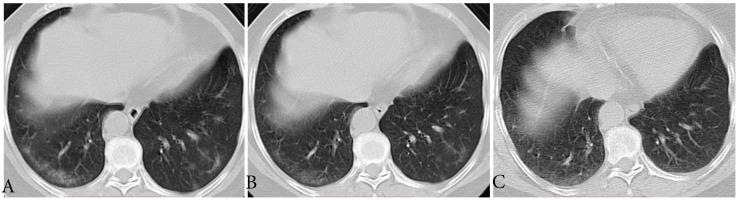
Typical evolution of type-GI in a 61-year-old female with asymptomatic SARS-CoV-2 infection. A, Day 0, the first chest CT showed multifocal lesions of subpleural GGO in bilateral lower lobe. B, Day 3, obvious resolution of the first GGO was observed. C, Day 6, continued resolution with minimal residual GGO was observed, the patient had two consecutive negative results of RT-PCR (day 6 and 7). GGO, ground-glass opacity; GI, gradual improvement; SARS-CoV-2, severe acute respiratory syndrome coronavirus 2.
